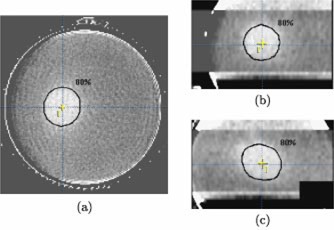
(Color) (a) Axial, (b) coronal, and (c) sagittal CT images of PAG2 (irradiated to a maximum dose of 8 Gy) at isocenter. The coronal and sagittal images were reconstructed from a set of axial gel images (3 mm slice spacing). The planned 80% isodose contour and a cross indicating the isocentre are marked in each image.
